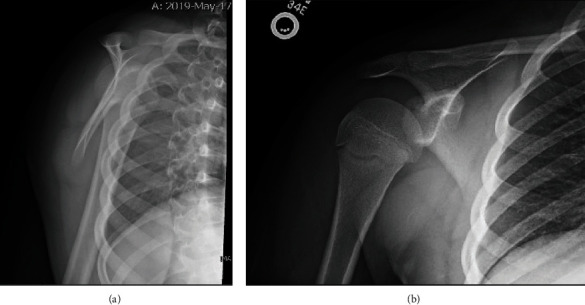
(a) Scapular X-ray taken during patient's first visit to emergency department. (b) Anteroposterior X-ray taken during patient's first visit to emergency department.
